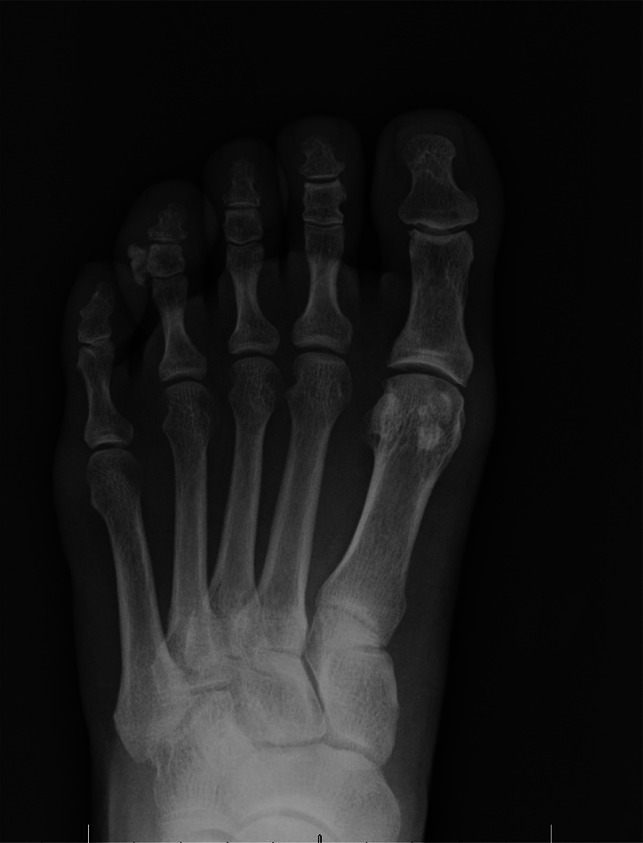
Radiograph depicting gouty tophus and deformity of the fourth toe middle phalanx (author's clinical photograph).
